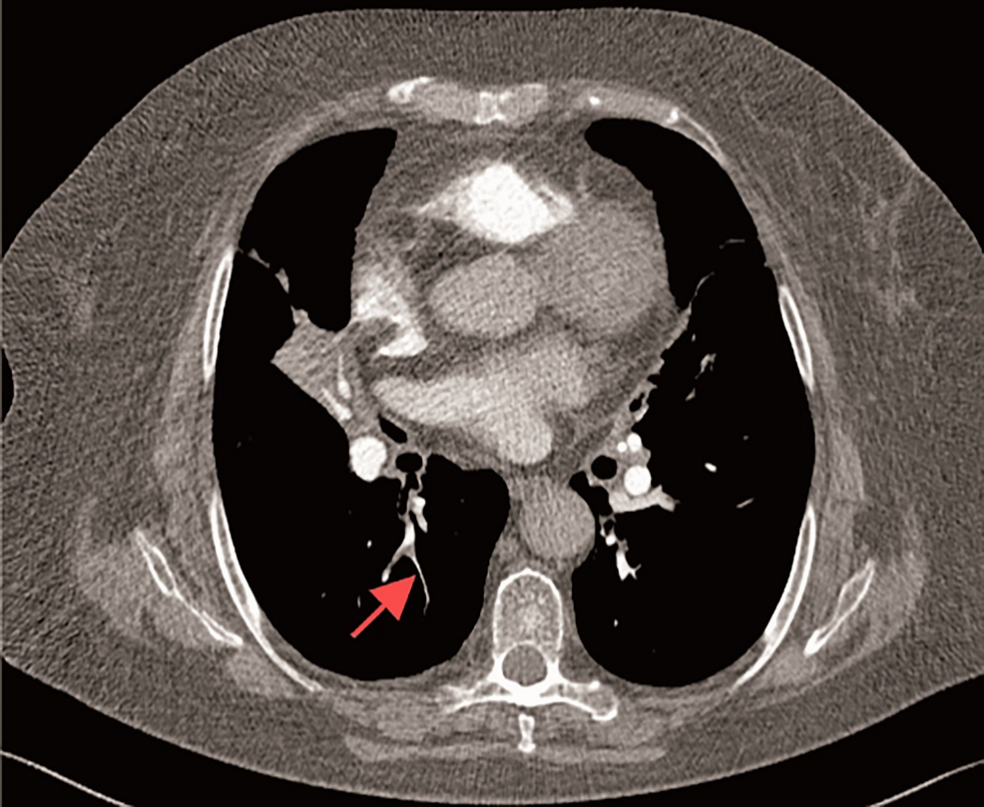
The patient's computed tomography pulmonary angiography (CTPA) revealed a low-density filling defect in the pulmonary artery within the dorsal segment of the right lower lobe of the lung, indicative of chronic pulmonary embolism (red arrow).
